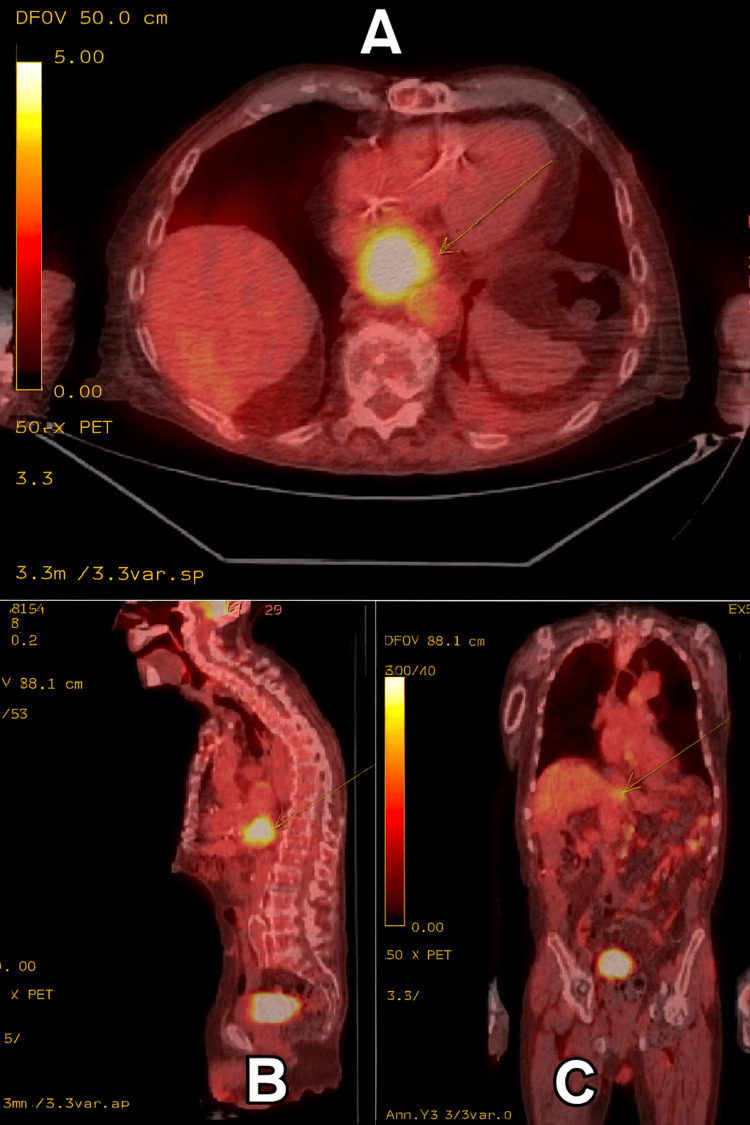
Axial (A), sagittal (B), and coronal (c) view of 18FDG-PET scan of the patient with arrows showing uptake limited to the distal esophagus 18F-FDG: fluorodeoxyglucose
